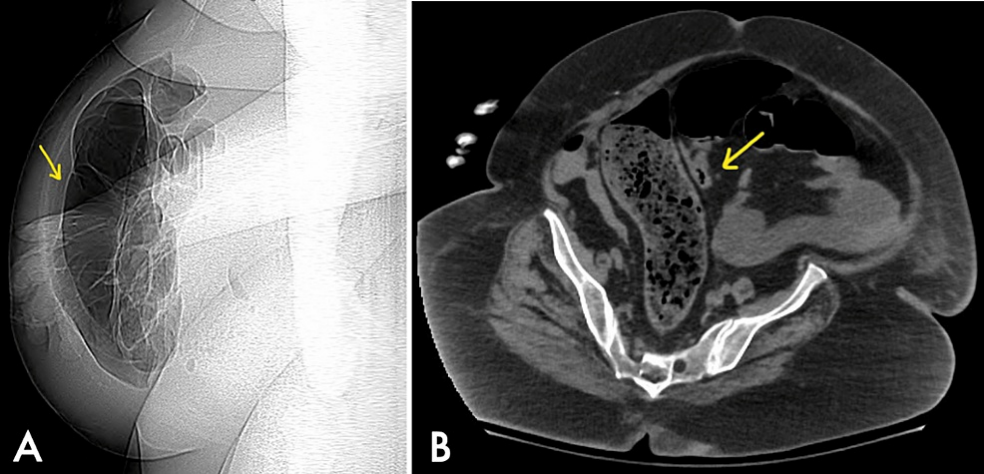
Abdomen/pelvis computed tomography scan: scout film with the yellow arrow showing marked bowel dilation (A), and axial view with the yellow arrow pointing at the transition area of sigmoid volvulus (B)
